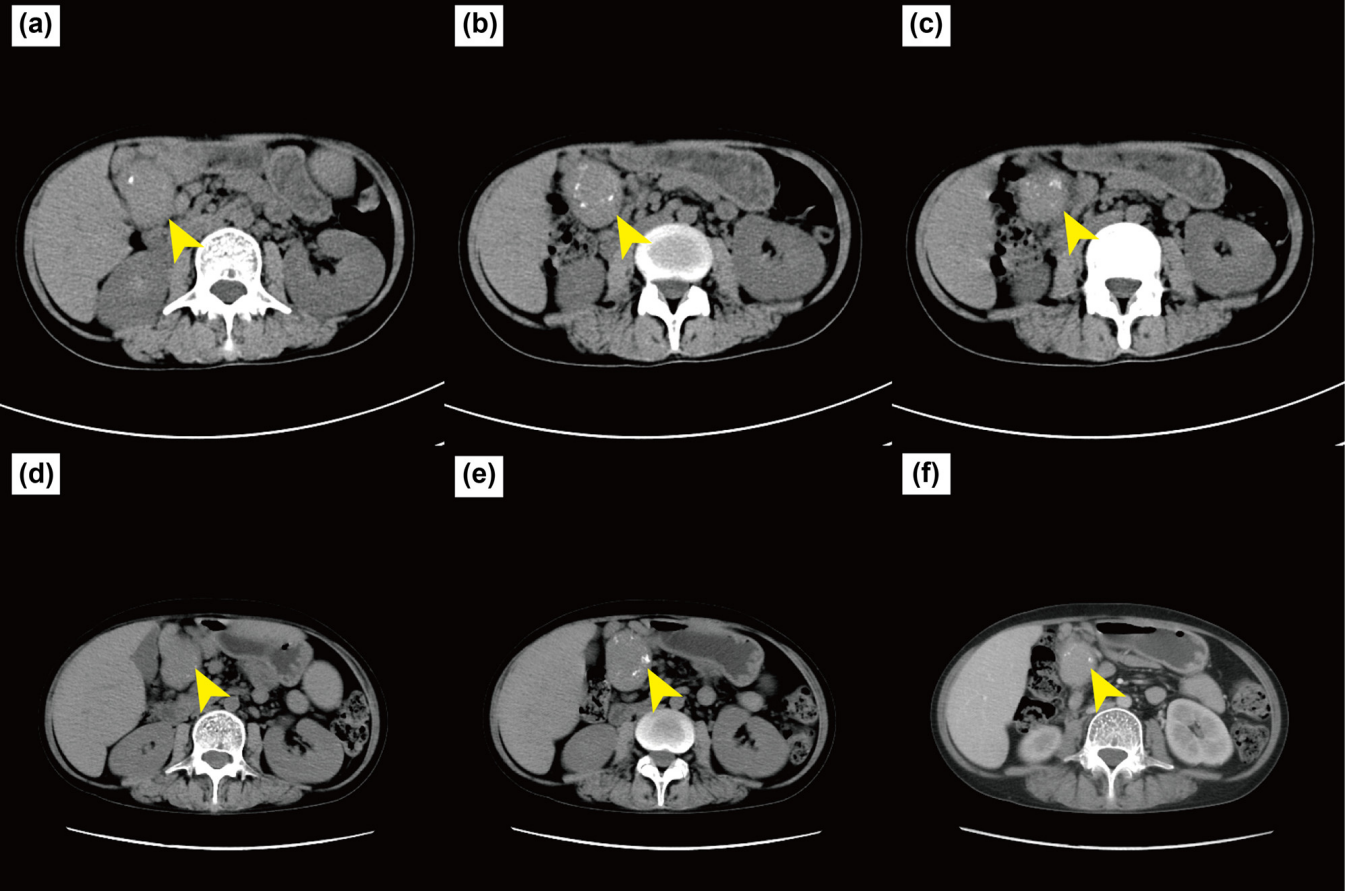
Abdominal computed tomography. Before (a)–(c) and after (d)–(f) chemotherapy axial; yellow arrow, upper left mass.
